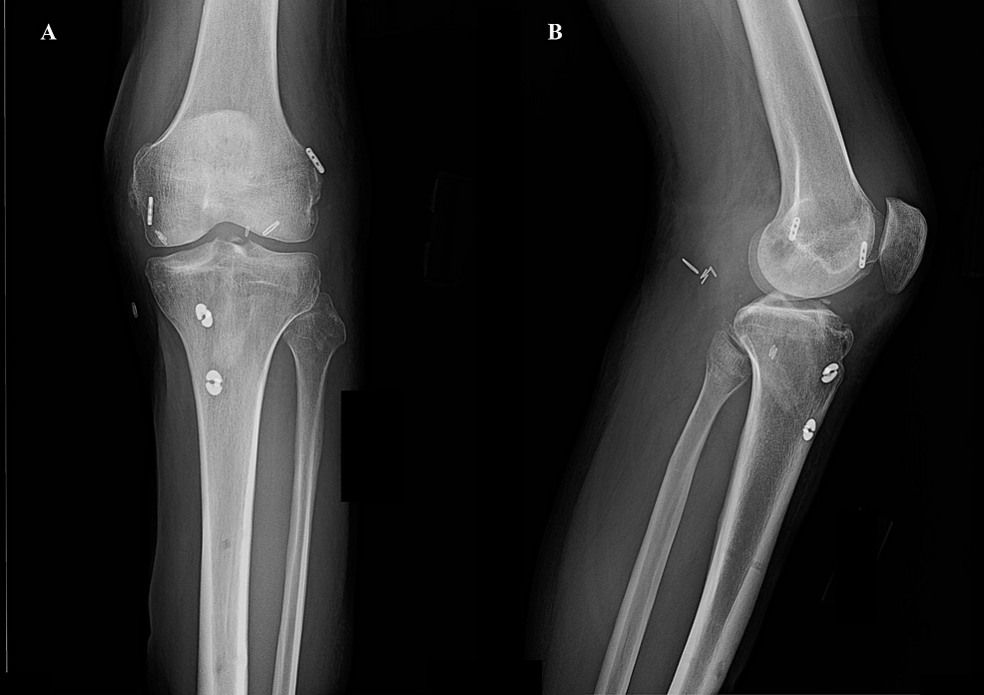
Left knee postoperative radiographs. Final left knee postoperative radiographs demonstrating proper graph fixation placement in the anterior-posterior (A) and lateral (B) views. The vascular clips from the popliteal artery repair can be seen in the lateral image (B).
